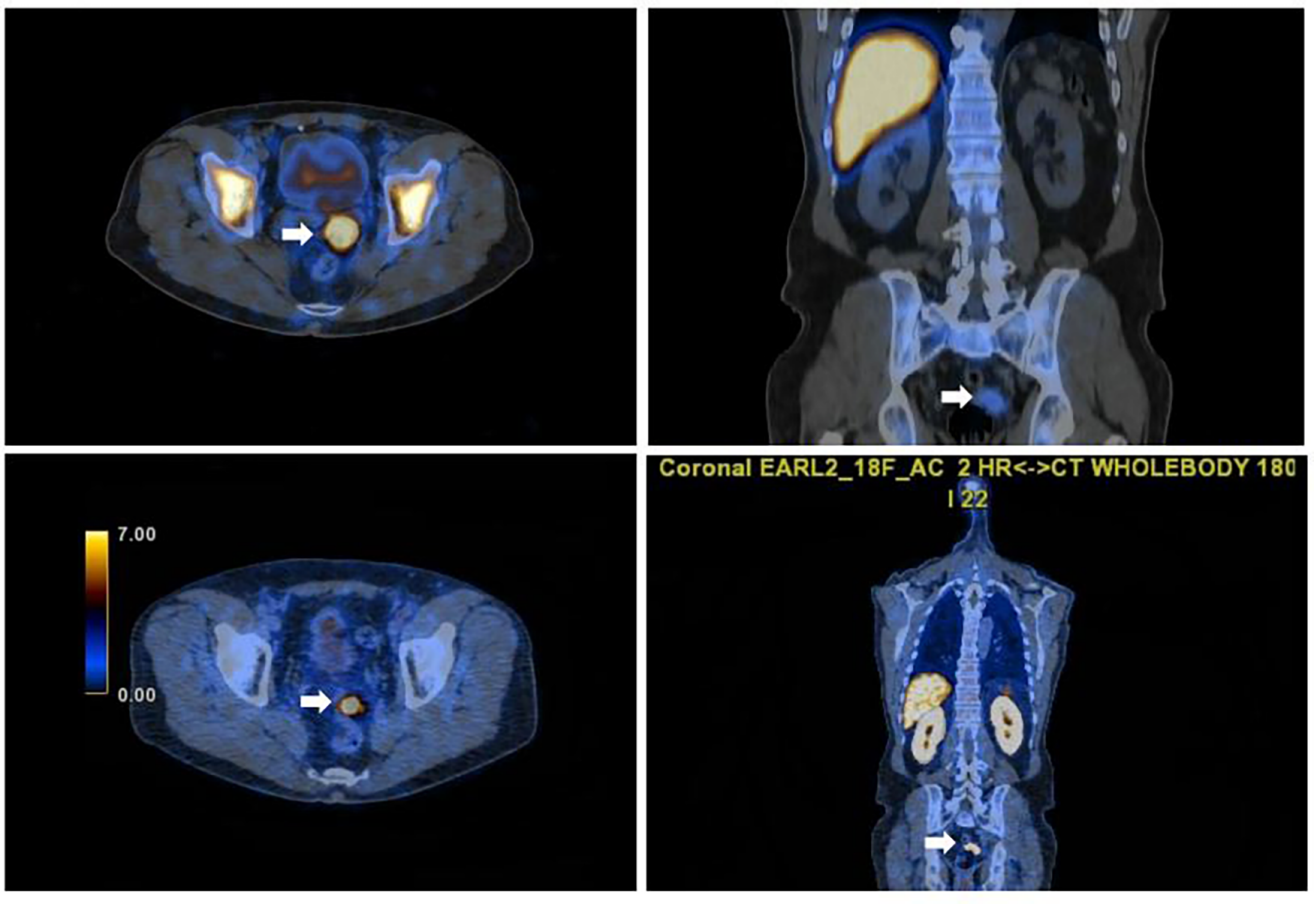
Axial and sagittal fused SPET/CT images of Tc-99m scan(left and right pictures) confirming functioning pelvic splenic tissue and concordant with the focal PSMA uptake seen on the PSMA PET/CT images (right and left lower images).
